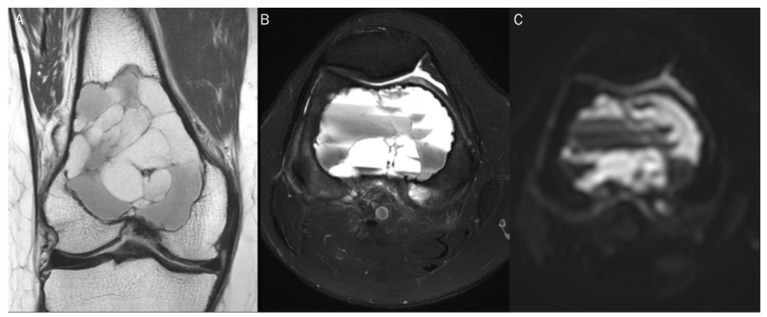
A 60-year-old female with giant-cell tumor with secondary aneurysmal bone cyst change in the distal femur. (A) Coronal T2-weighted image and (B) axial T2-weighted fat-suppressed image show secondary aneurysmal bone cyst change with fluid–fluid level. (C) The DWI with *b*-value of 0 s/mm^2^ appears similar to the heavily T2-weighted fat-suppressed image (B).
